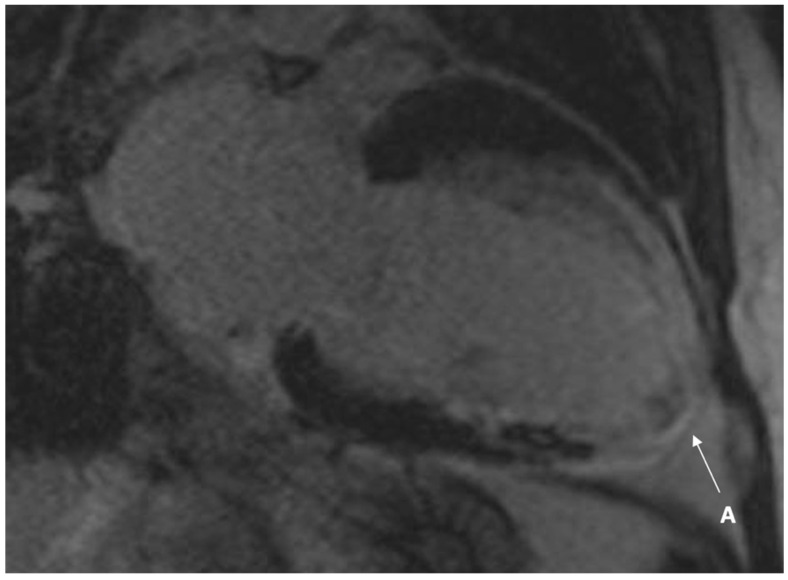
Cardiac magnetic resonance imaging (CMR) vertical long-axis view demonstrating extensive apical transmural late gadolinium enhancement in a region with myocardial thinning and akinesis, consistent with completed myocardial infarction (A).
